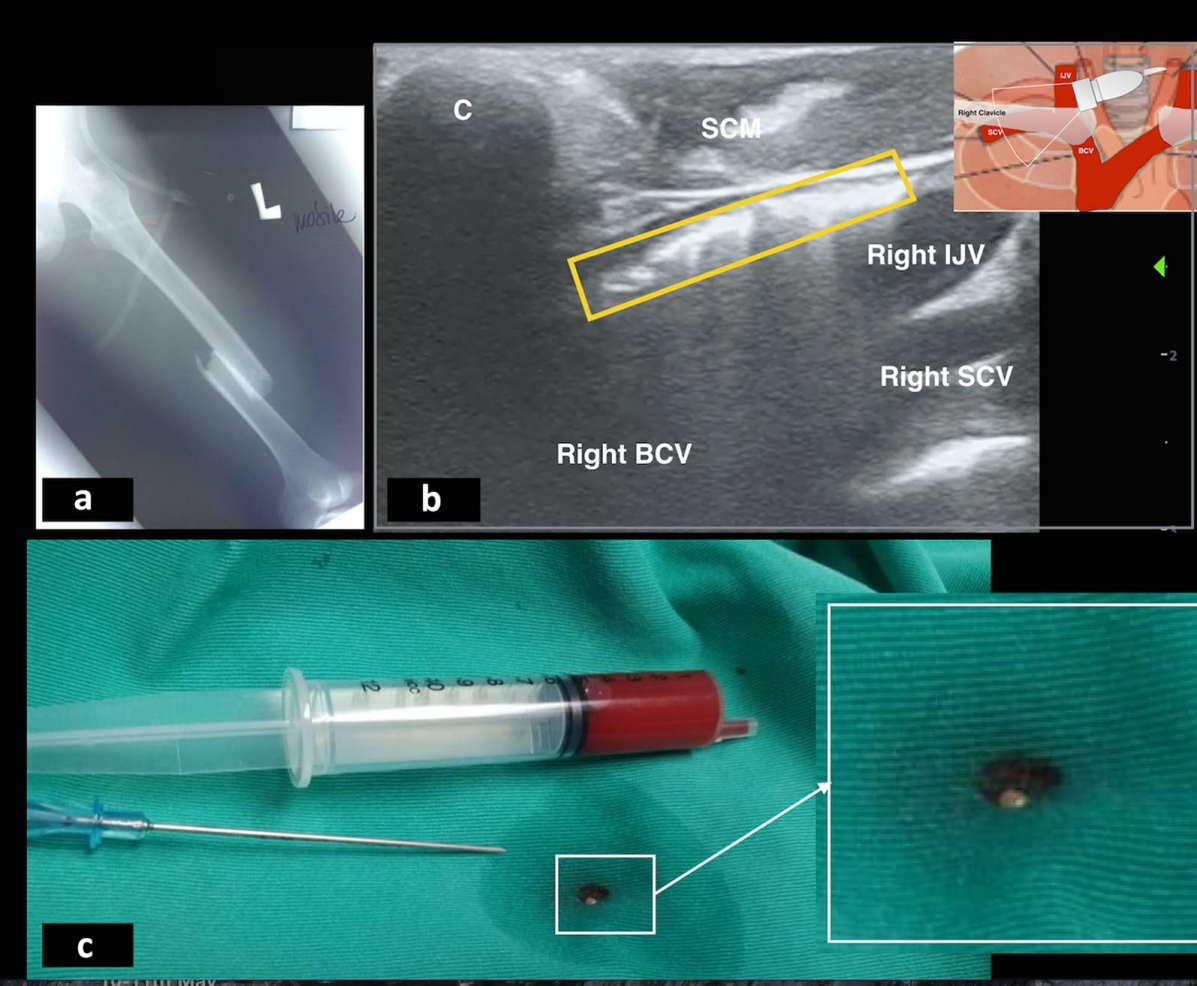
a X-ray showed closed fracture right femoral shaft. b Ultrasound images revealed free floating mobile hyperechoic spots, located in the anterior part of the vein and cleared from the vein (yellow box). Small picture showing probe location in relation to clavicle, internal jugular vein, subclavian vein and brachiocephalic vein. c Fat globule was aspirated during cannulation of the IJV as shown in the white box. *C* Clavicle, *IJV* internal jugular vein, *SCV* subclavian vein, *BCV* brachiocephalic vein
